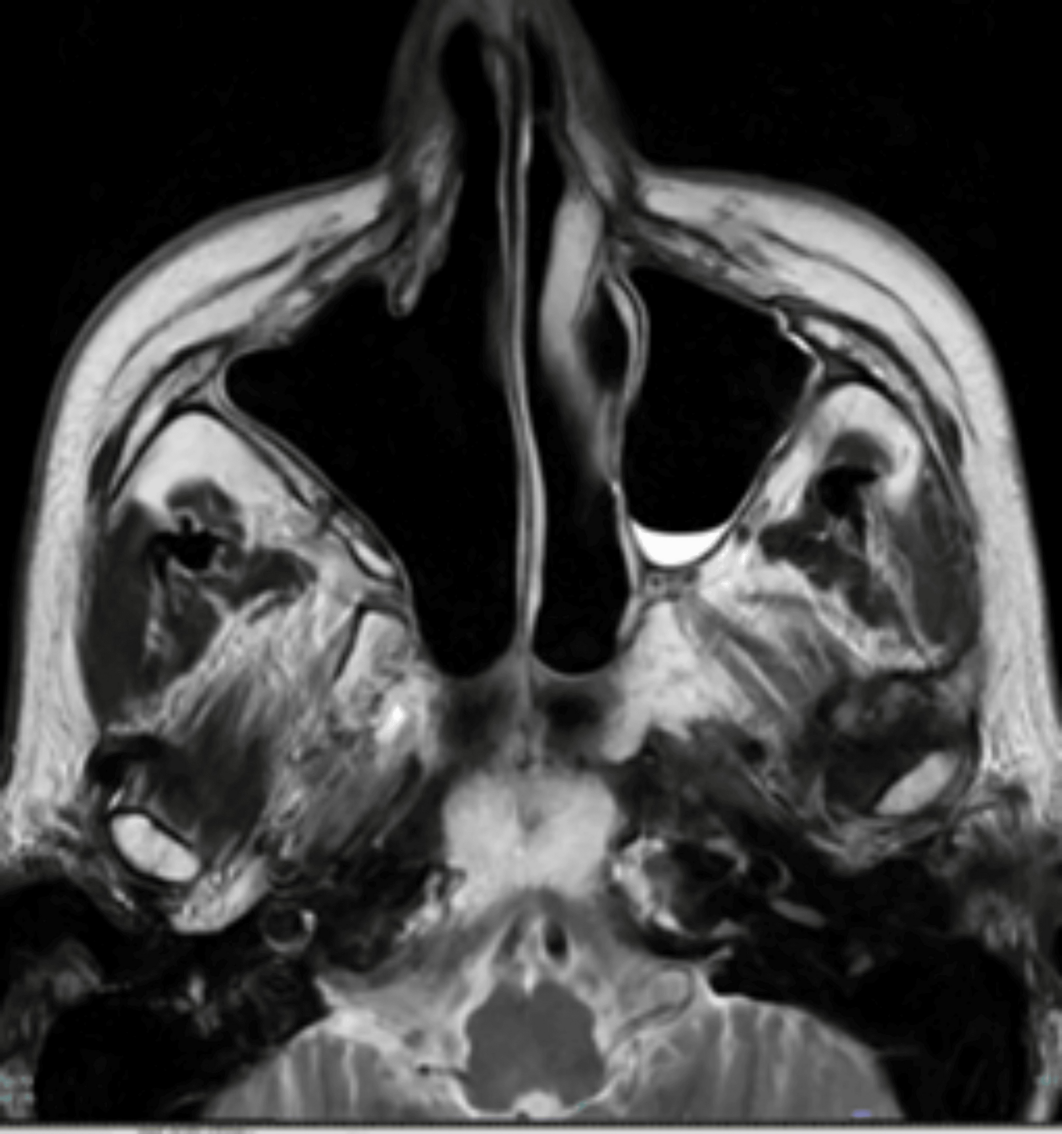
Post-gadolinium T1 weighted MRI in February 2025 (axial view) showing maintained complete local response
